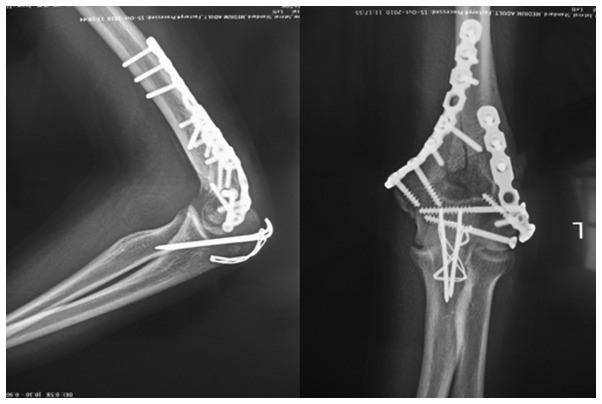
Postoperative radiographs following Y-shaped plating fixation for distal humerus fractures.
